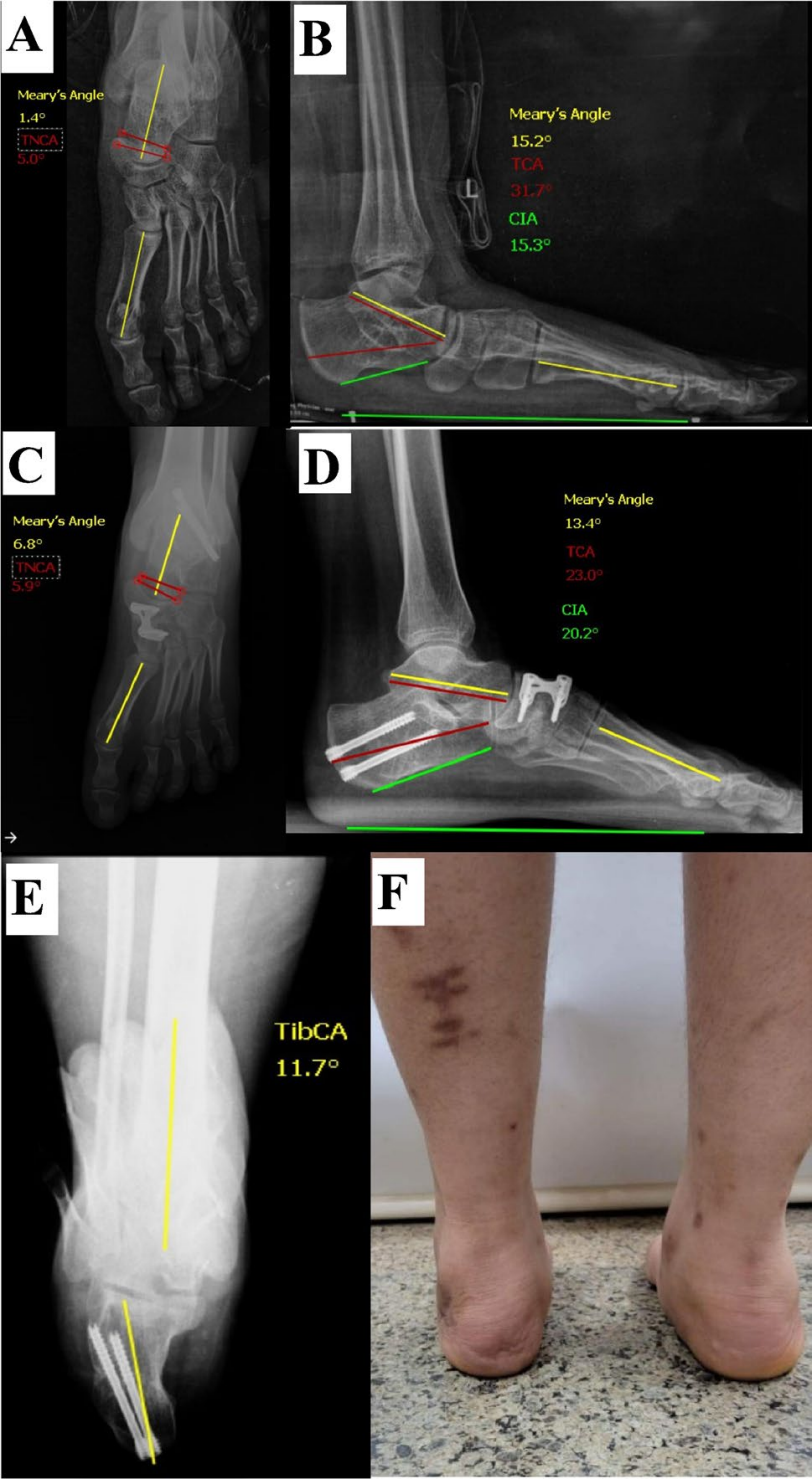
A female patient, 15 years old, had an MDCO combined with a naviculo-cuneiform fusion. A, B Preoperative radiographs. C–E Last follow-up (16 months) radiographs showing deformity correction (as per angles measured) and restoration of the heel alignment as shown in the axial view. F A clinical image showing restoration of the heel alignment
